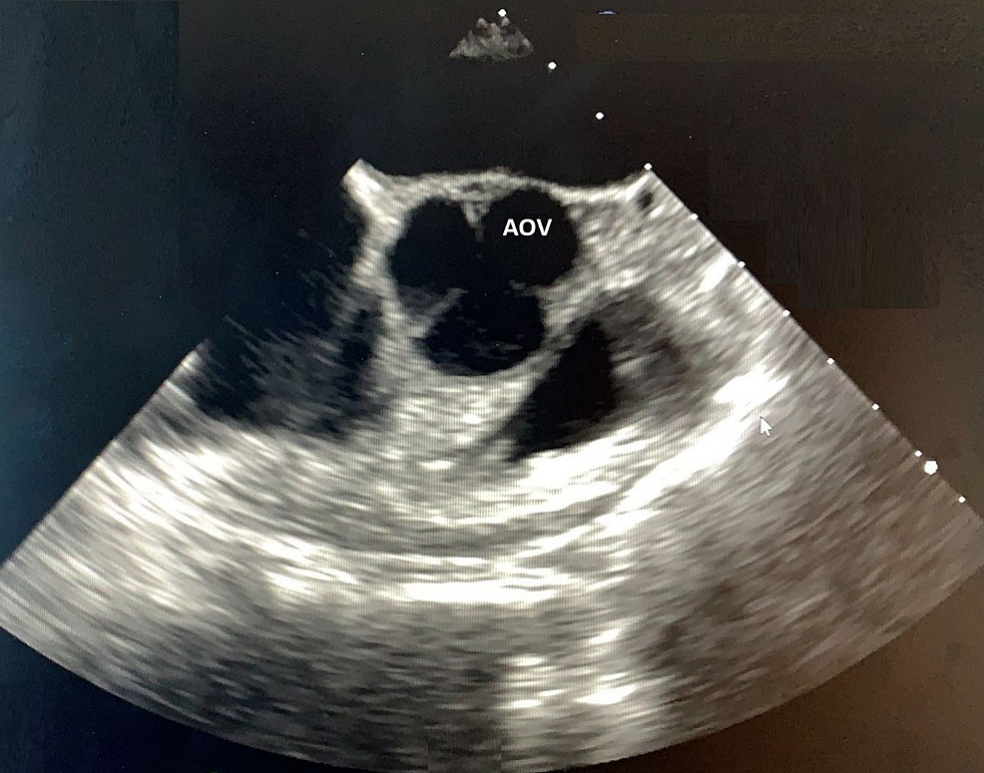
Transesophageal echocardiogram evaluating the aortic valve. AOV: aortic valve.
